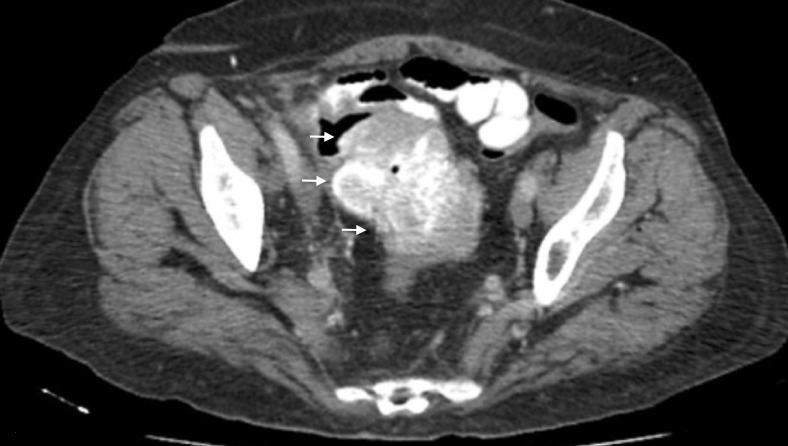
The sagittal cross section from the computed tomography (CT) of the abdomen and pelvis, performed in 2016, of the ovarian mass.
